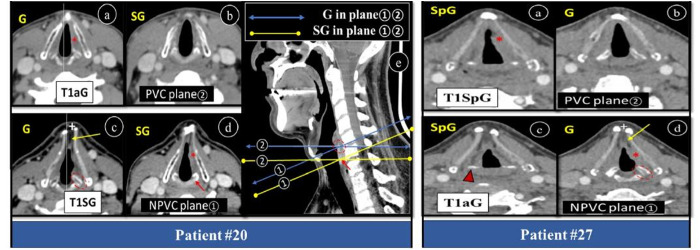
Axial and sagittal post-contrast CT larynx showing change in T1 tumor origin due to CT plane. Patient # 20: Axial (a-d) and sagittal (e) post-contrast CT larynx showing change in location of T1aG tumor PVC plane to T1SG in NPVC plane. Plane② (a,b) showing left VC origin, while plane① (c,d) left SG origin. Asterisks (*) showing areas of tumor extension (G, SG), dotted oval (c,e) is CAJ at glottic level, arrow is at cricoid cartilage of SG. Sagittal plane (e) is pictorial demonstration of reason for tumor location change. Patient #27: Axial post-contrast CT larynx showing change in location of T1 SpG in PVC plane to T1aG in NPVC plane. Plane② (a,b) showing left false cord origin, while plane① (c,d) left true VC origin. Asterisks (*) showing areas of tumor extension (SpG, G), arrowhead is upper part of AC of SpG level, dotted oval is CAJ at glottic level. Also note false anatomical landmarks of G in NPVC plane, i.e., fat (long yellow arrow) and open thyroid notch (+) in both patients. *SpG = supraglottis, G = glottis, NPVC = non-parallel vocal cord, PVC = parallel vocal cord, AC = arytenoid cartilage, CAJ = cricoarytenoid joint.*
